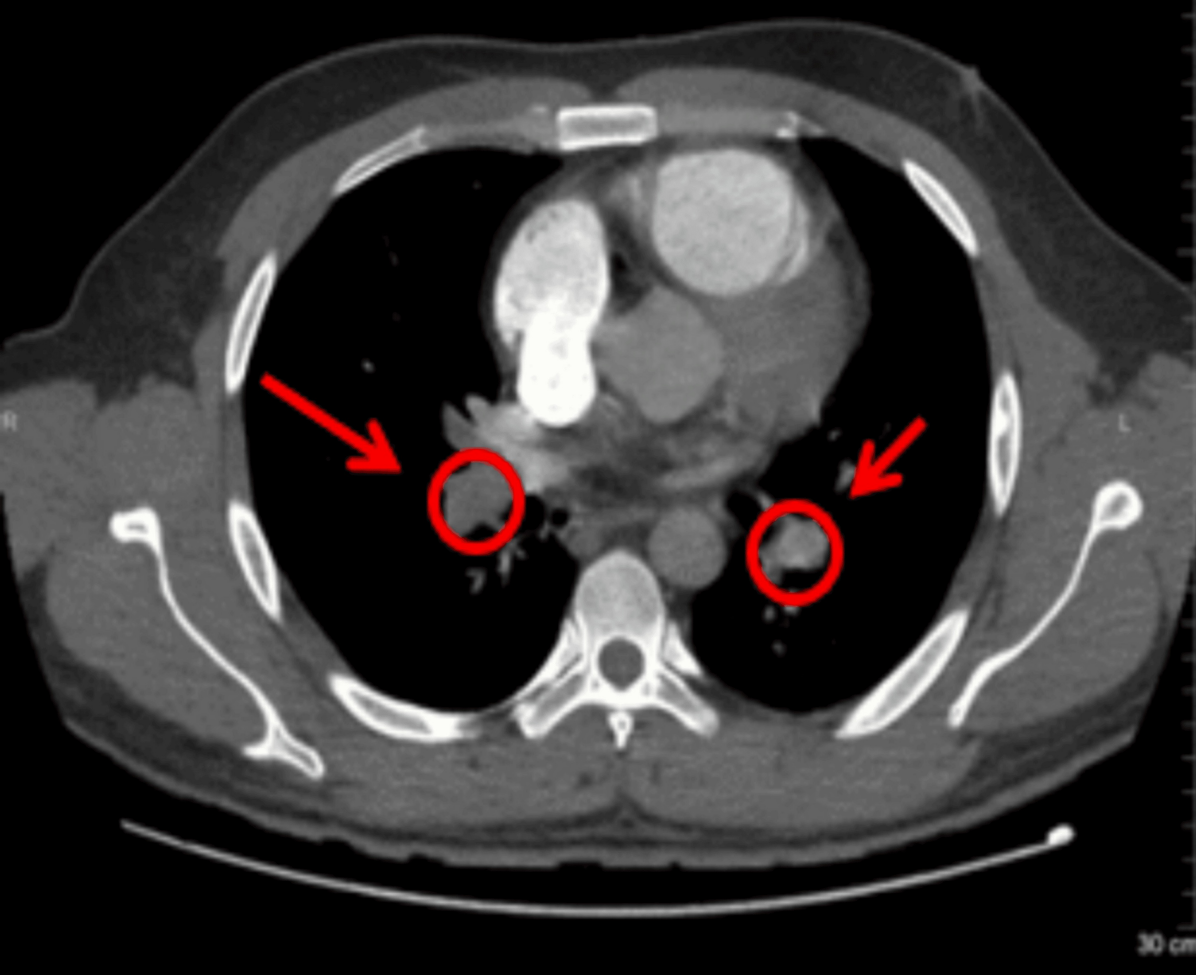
The CT pulmonary angiogram demonstrates a filling defect within the right and left pulmonary arteries, consistent with a massive bilateral pulmonary embolism The thrombus is visible as an area of low attenuation in the main pulmonary arteries, showing obstruction of blood flow.
